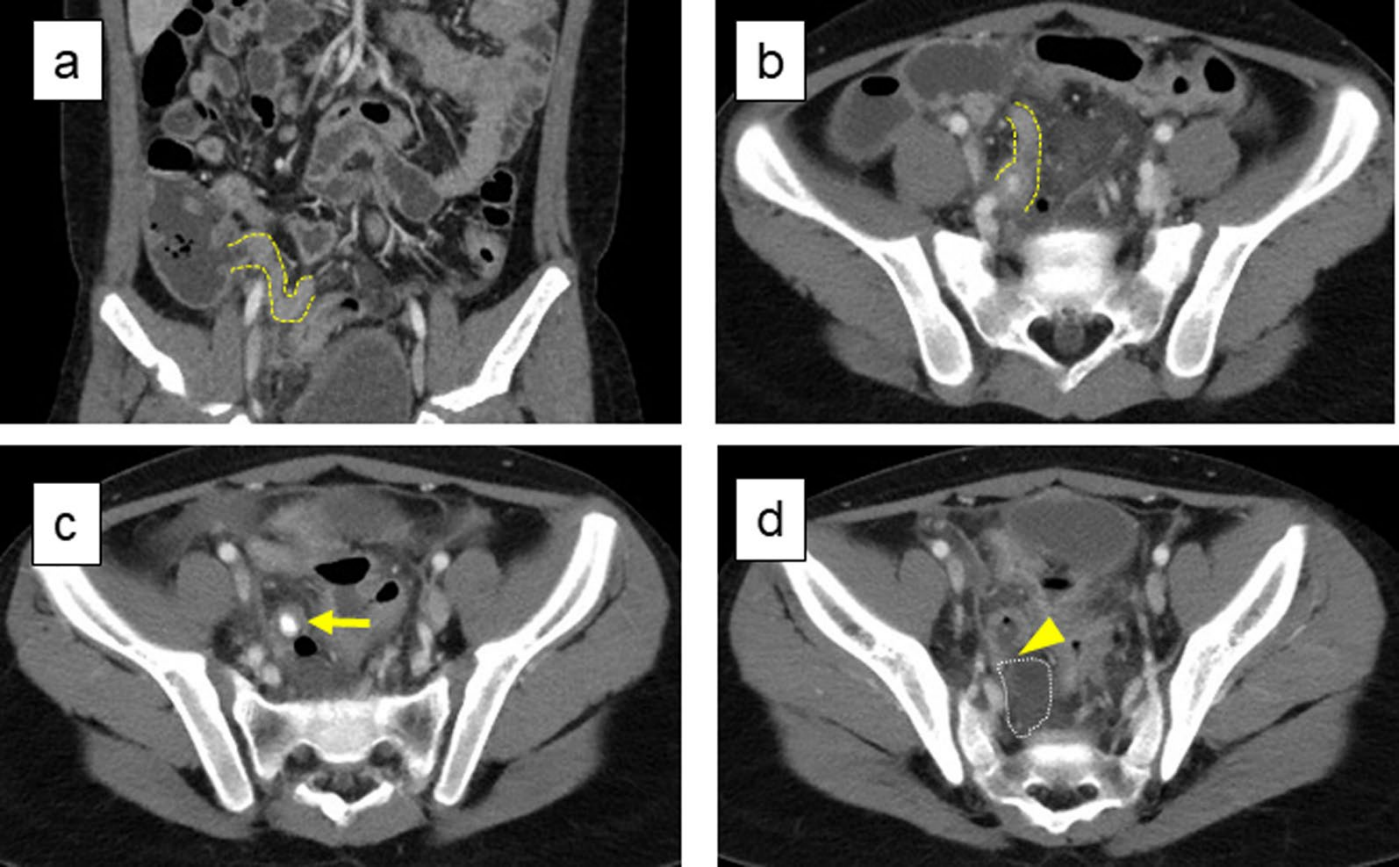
Enhanced computed tomography on admission. CT showed the swollen appendix with an intact appendicular base (a) and body (b), with a fecalith in the lumen (c), and perforation on the apex side (d), accompanied by fluid collection in the peri-appendiceal region (d). The yellow dotted line shows the appendicular body. The yellow arrow indicates a fecalith in the lumen. The yellow arrowhead shows the point of perforation. The white dotted line shows fluid collection in the peri-appendiceal region
